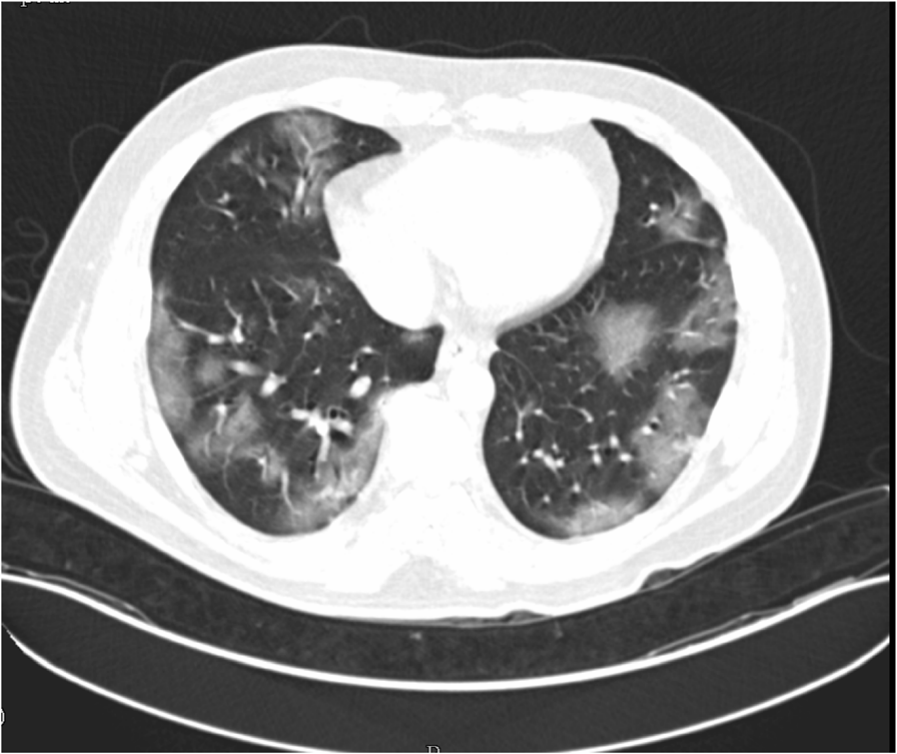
Chest CT scan showing multiple bilateral and peripheral ground-glass and consolidative pulmonary opacities
